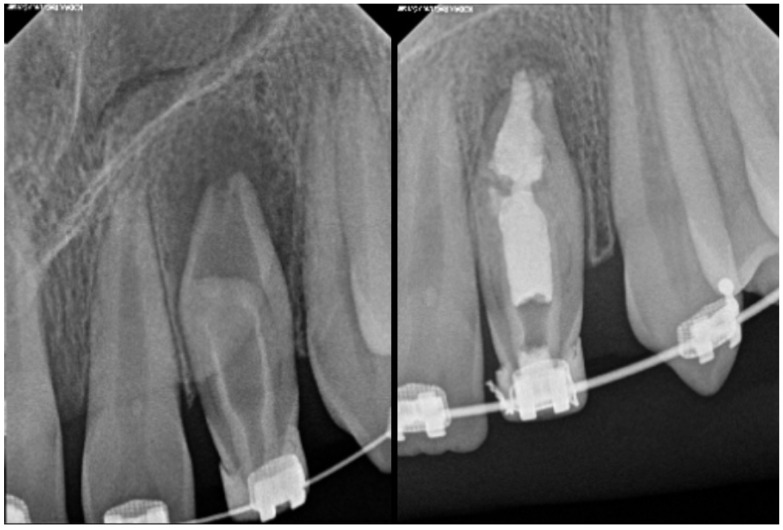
Radiographic features of Oehlers Type II *dens invaginatus* case in lateral incisor before (left) and after (right) treatment (courtesy of C.A.).
